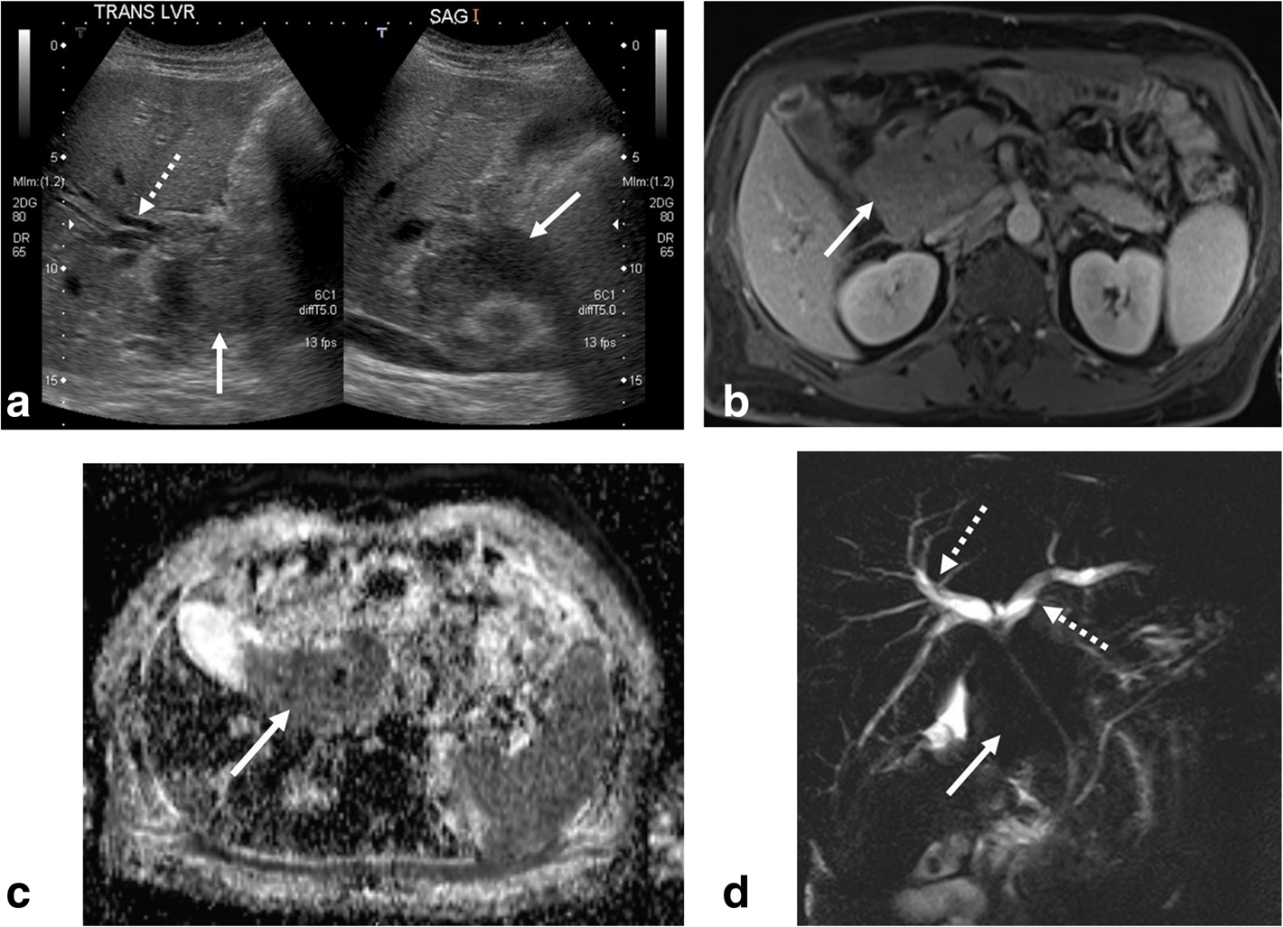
Nodal mass with biliary obstruction. A 55-year-old male presenting with jaundice. a Initial grayscale transverse and sagittal ultrasound images demonstrate an ill-defined hypoechoic mass near the porta hepatis (solid arrow) with associated biliary dilatation. Follow-up MRI with (b) axial post contrast, (c) apparent diffusion coefficient map, and (d) coronal MRCP images demonstrate an enhancing mass with diffusion restriction (solid arrows) encasing and narrowing the extrahepatic bile ducts causing intrahepatic biliary dilatation (dashed arrow)
